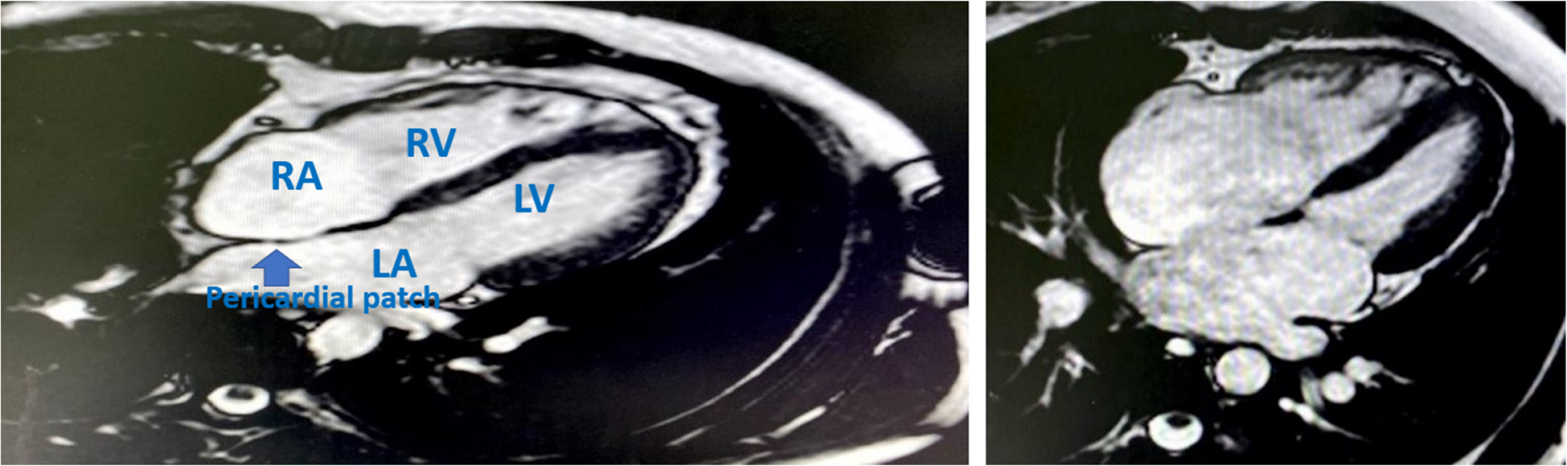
Cine SSFP 4 chamber MRI view in surgically closed case by conventional method with revolution of RA and RV volumes
